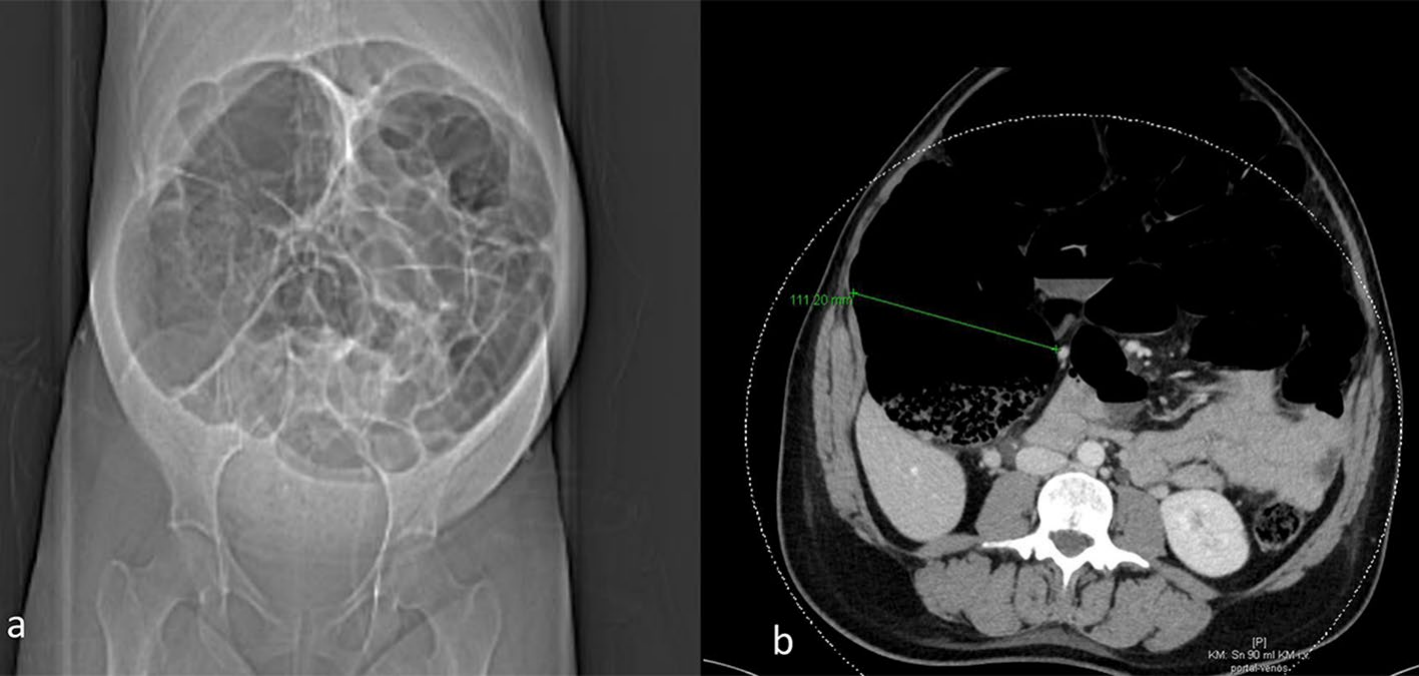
a Abdominal X-ray. Extremely distended abdomen with signs of massive dilatation of the colon. b CT massive dilatation of colon especially caecum up to 11 cm (marked with a green arrow)
